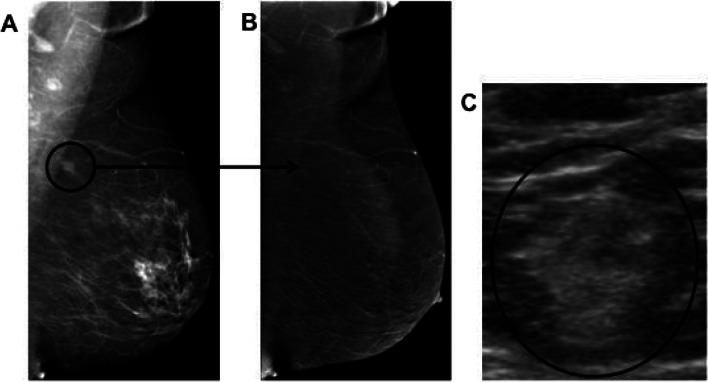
Example of lack of enhancement due to small size. Patient was referred from an outside institution for abnormal screening mammogram. A CEM shows a 1.1 cm spiculated mass (black circle) on the LE image in the posterior left upper breast, axillary tail region, only seen on the MLO view. B No enhancement is seen on RI. C Subsequent ultrasound revealed an indistinct 1.2 cm echogenic mass (black circle) which correlated with the mammogram finding. Ultrasound guided biopsy confirmed invasive ductal carcinoma
